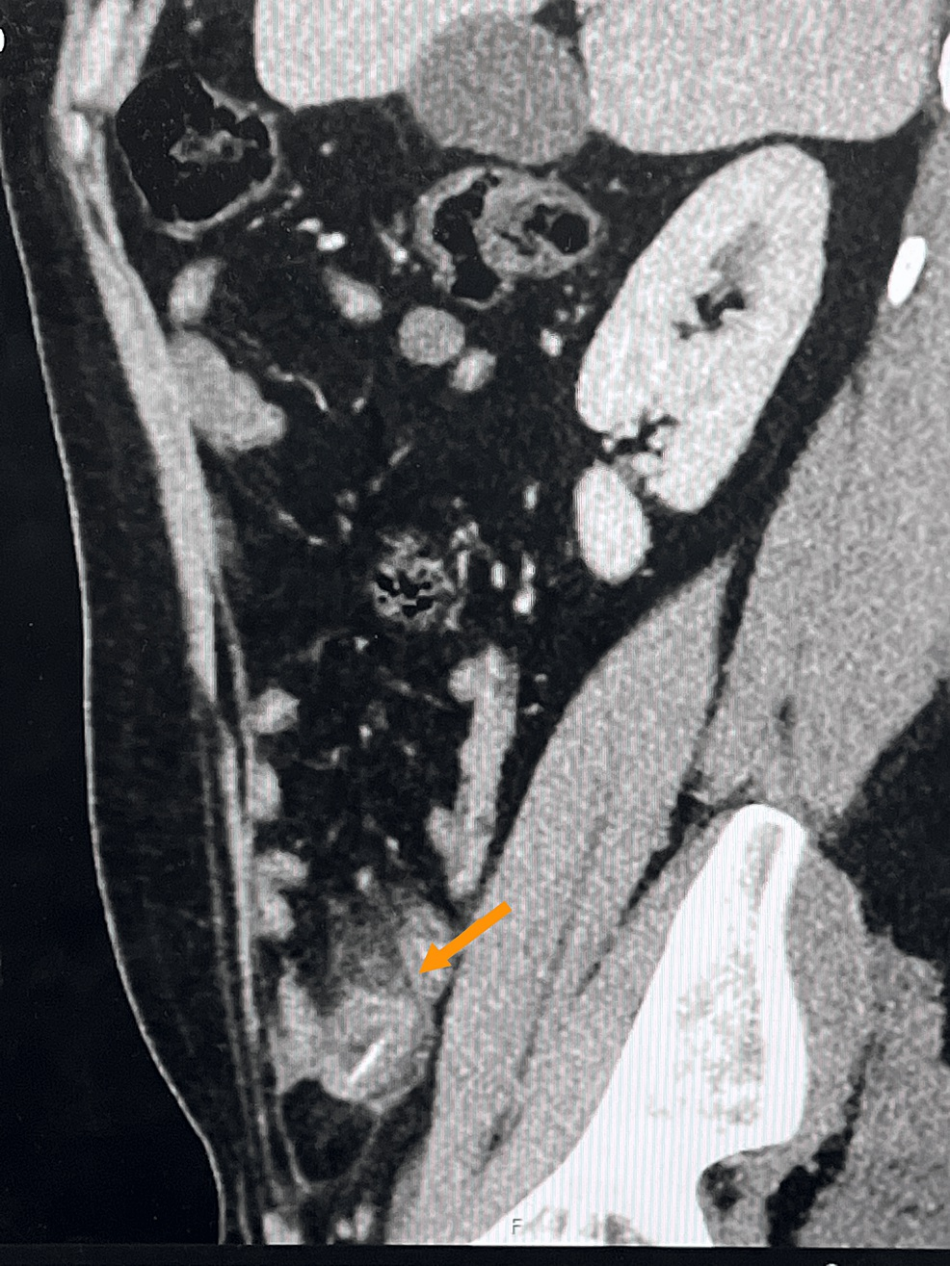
A sagittal cut section of the CT abdomen and pelvis demonstrates a perforated Meckel's diverticulum.
